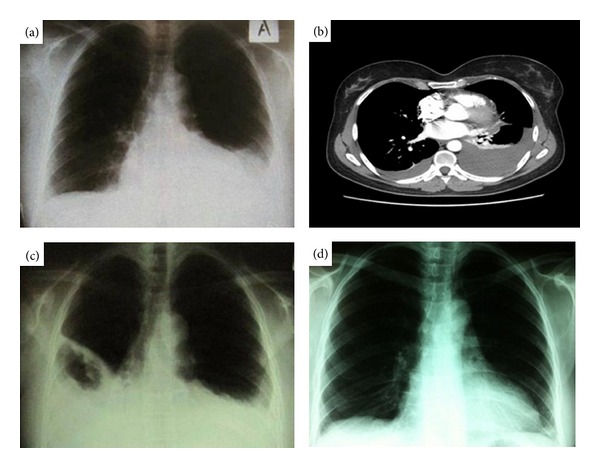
(a) Chest X-ray at presentation showing left-side pleural effusion. (b) CT pulmonary angiography showing bilateral pleural effusion. (c) Chest X-ray at the fifth day. (d) Chest X-ray at the third month.
